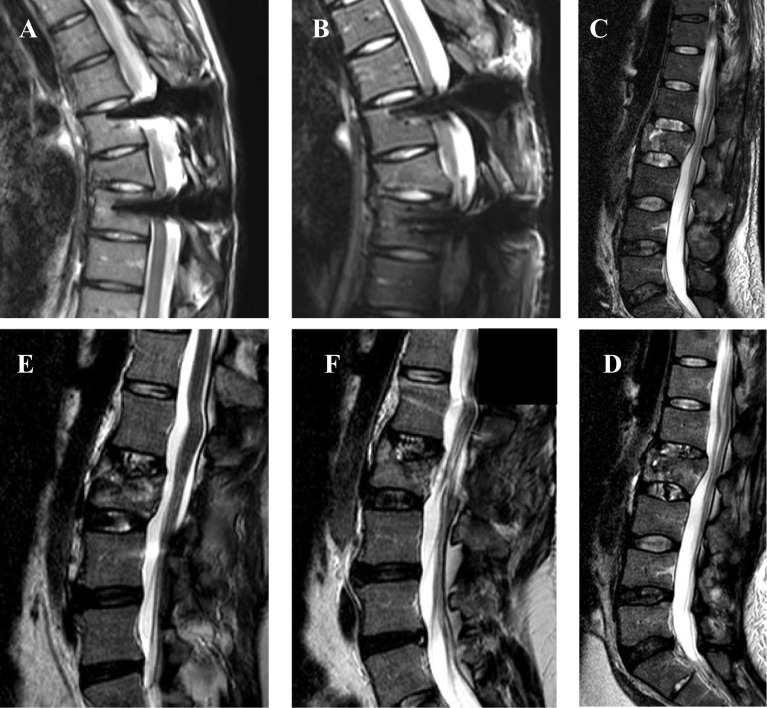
A and B: T2-weighted sagittal MRI images of a T7 fracture (A: post-traumatic and B: at follow-up) showing Oner I and Pfirrmann I adjacent discs. C and D: T2-weighted sagittal MRI images of a L2 fracture showing initial disc lesions (image C), with degeneration on both cranial and caudal discs at follow-up (image D). E and F: T2-weighted sagittal MRI images of a L2 fracture showing a Oner 3 lesion on the cranial disc.
